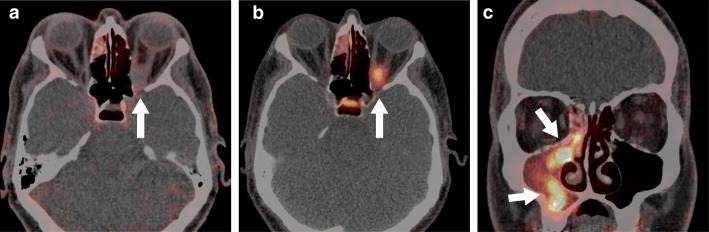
Patient with a meningioma in the left orbital apex and sinusitis of the right maxillary sinus (case 8). a Negative ^89^Zr-rituximab PET/CT (white arrow, SUVmax < 1.0). b Positive 68Ga DOTA-TATE PET/CT, white arrow. c
